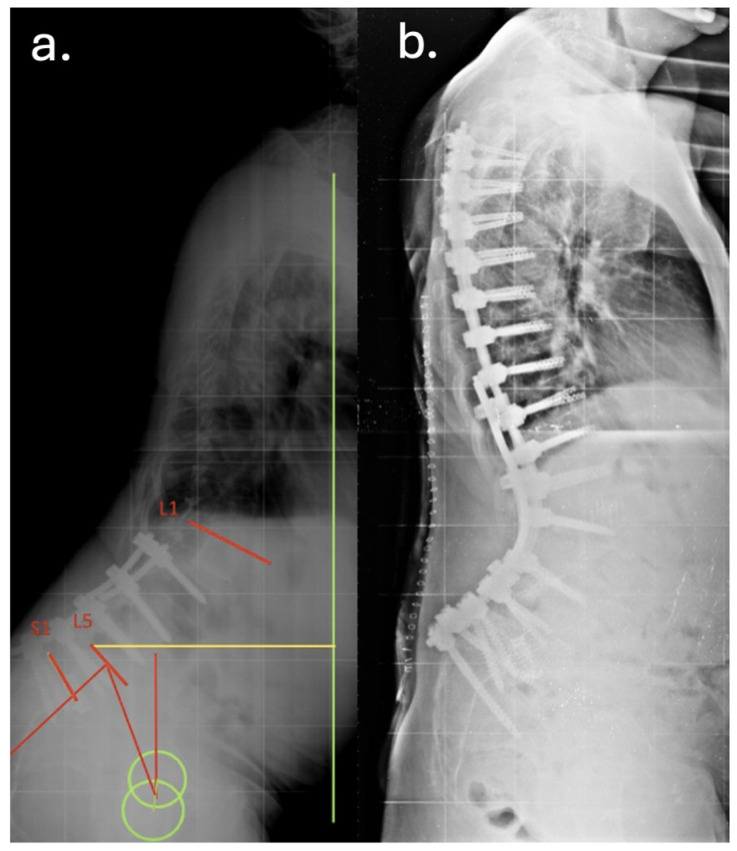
Preoperative (a) and postoperative (b) lateral radiographs documenting the first revision surgery with extension of the arthrodesis from T2 to the ilium. The images show restoration of sagittal alignment, with correction of the sagittal vertical axis (SVA) and improvement of lumbar lordosis.
